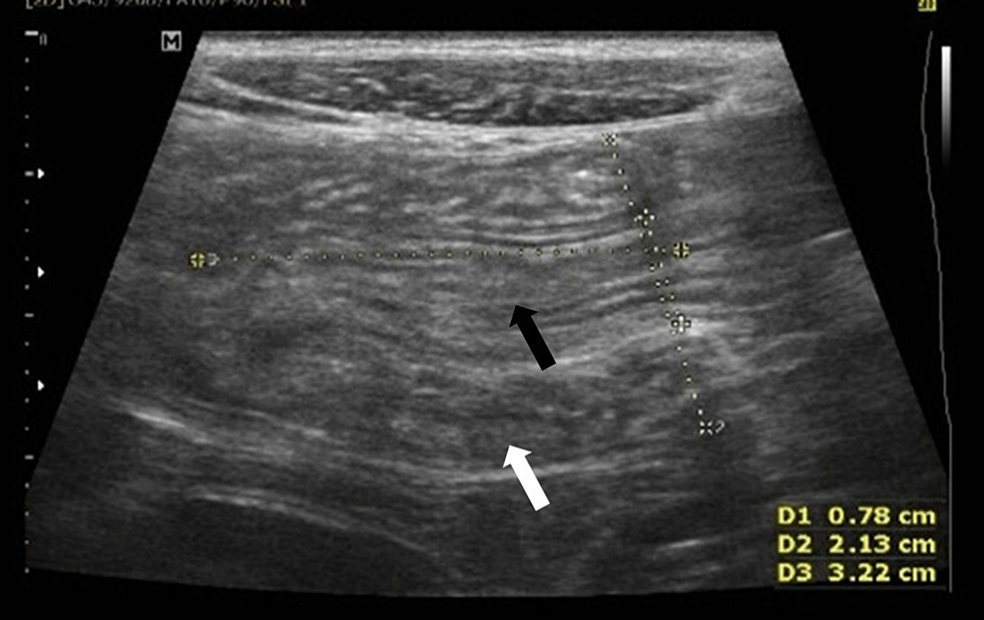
High-resolution sonographic view with a linear transducer placed in the right iliac fossa region in the longitudinal plane The image shows the telescoping of a segment of the small bowel loop (black arrow) into the large bowel (white arrow), suggestive of intussusception
